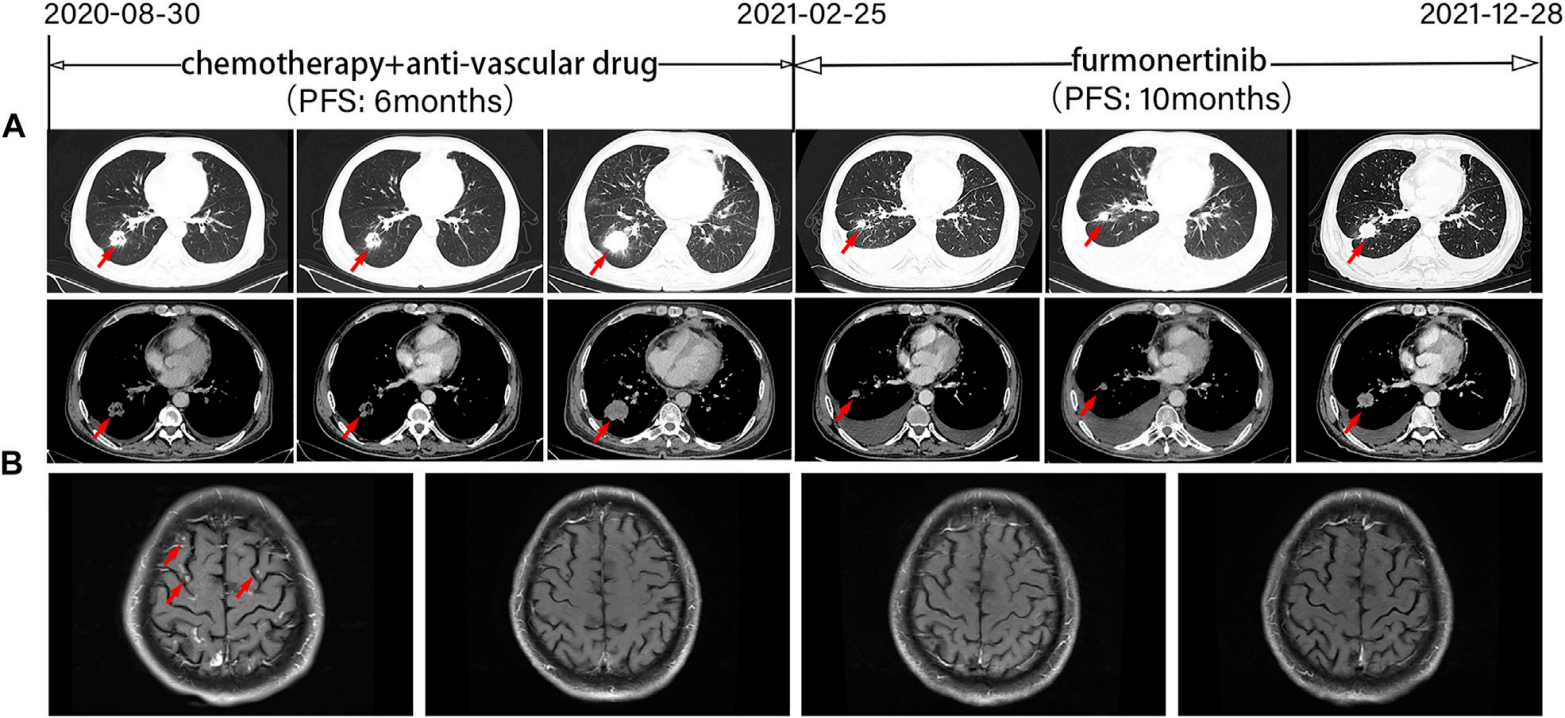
Tumor progression of the patient before and after treatment. (A) Representative computed tomography images at various points. CT images revealed lesions in the lower right lung. (B) Magnetic resonance images at various points. PFS, progression-free survival. The timeline of therapies, therapeutic regimens and tumor progression are indicated (top). The tumor is indicated by red arrows. SD, stable disease; PD, progressive disease; PR, partial response.
